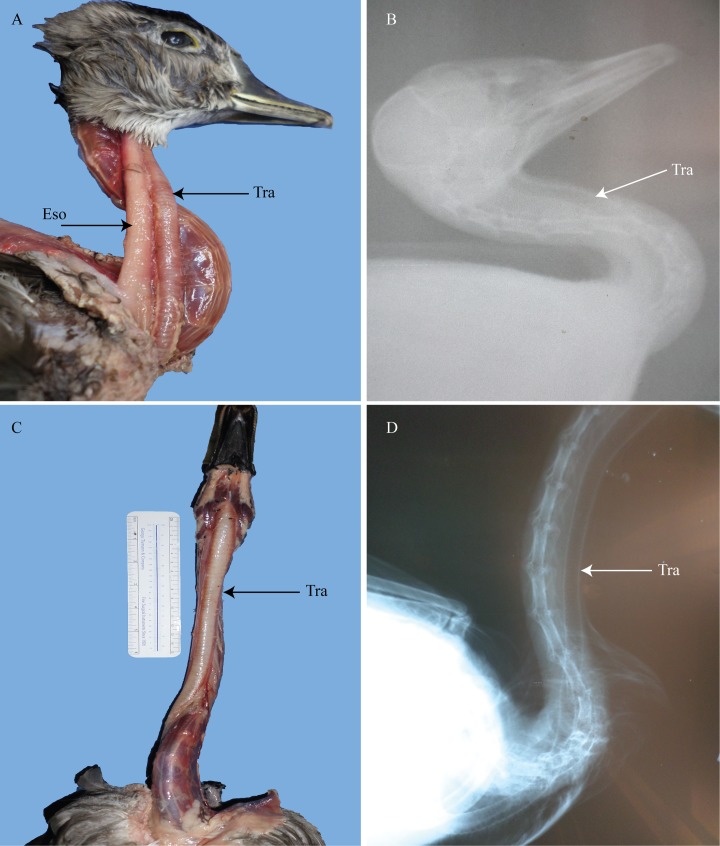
Duck and goose trachea and esophagus. (A) right lateral view of the neck of *Aix sponsa* showing displacement of the organs occurring quickly after exiting the oropharynx. (B) radiograph of *Aix sponsa*. (C) ventral view of the neck of *Branta canadensis* showing a gradual displacement of the organs so that they are lateralized only in the posterior region of the neck. (D) radiograph of lateral view of *Branta canadensis*. Tra = trachea; Eso = esophagus.
